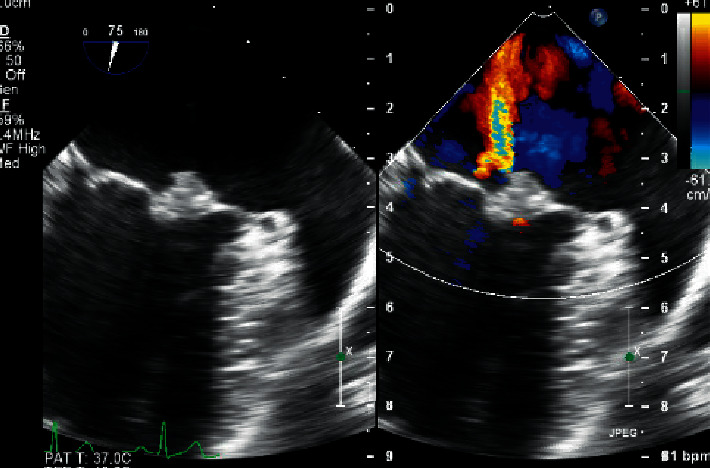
Echo view showing the left atrial mass with color Doppler.
